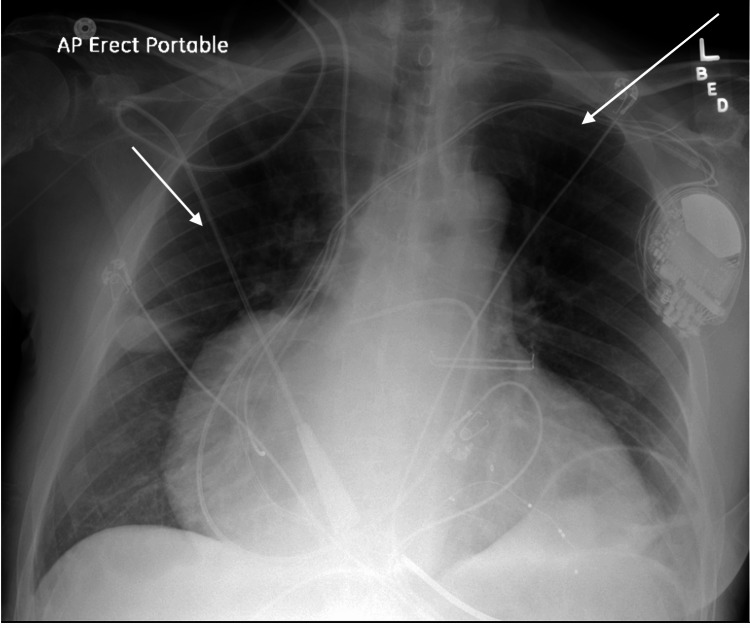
A chest X-ray showing mild pulmonary vascular congestion (white arrows)
